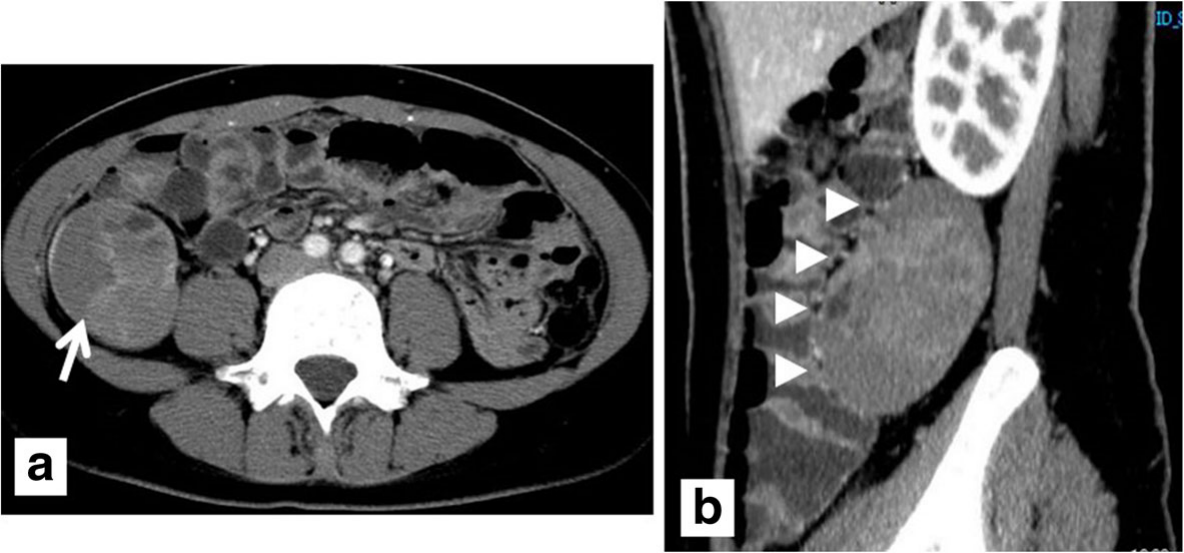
(a) Horizontal and (b) sagittal image of CT reveals a cystic and well-defined tumor 8.5 cm in diameter behind the ascending colon (arrow heads) with enhanced and segmented components (arrow)
